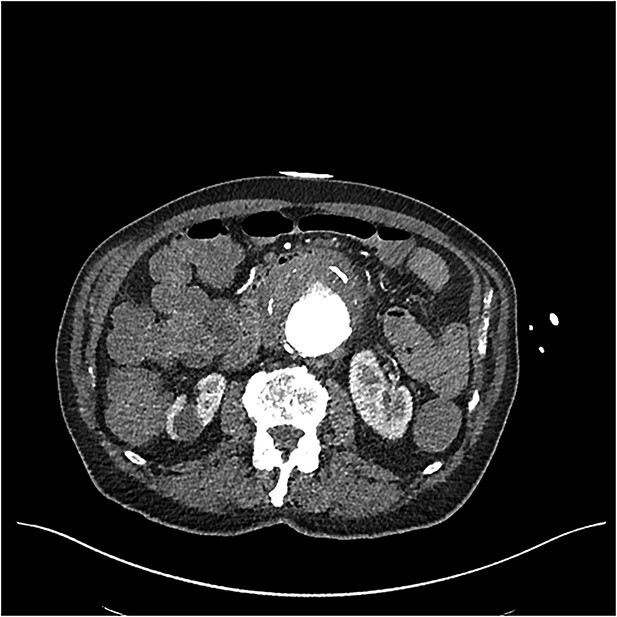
CT angiogram on initial presentation demonstrating an aneurysmal abdominal aorta but no contrast extravasation.
